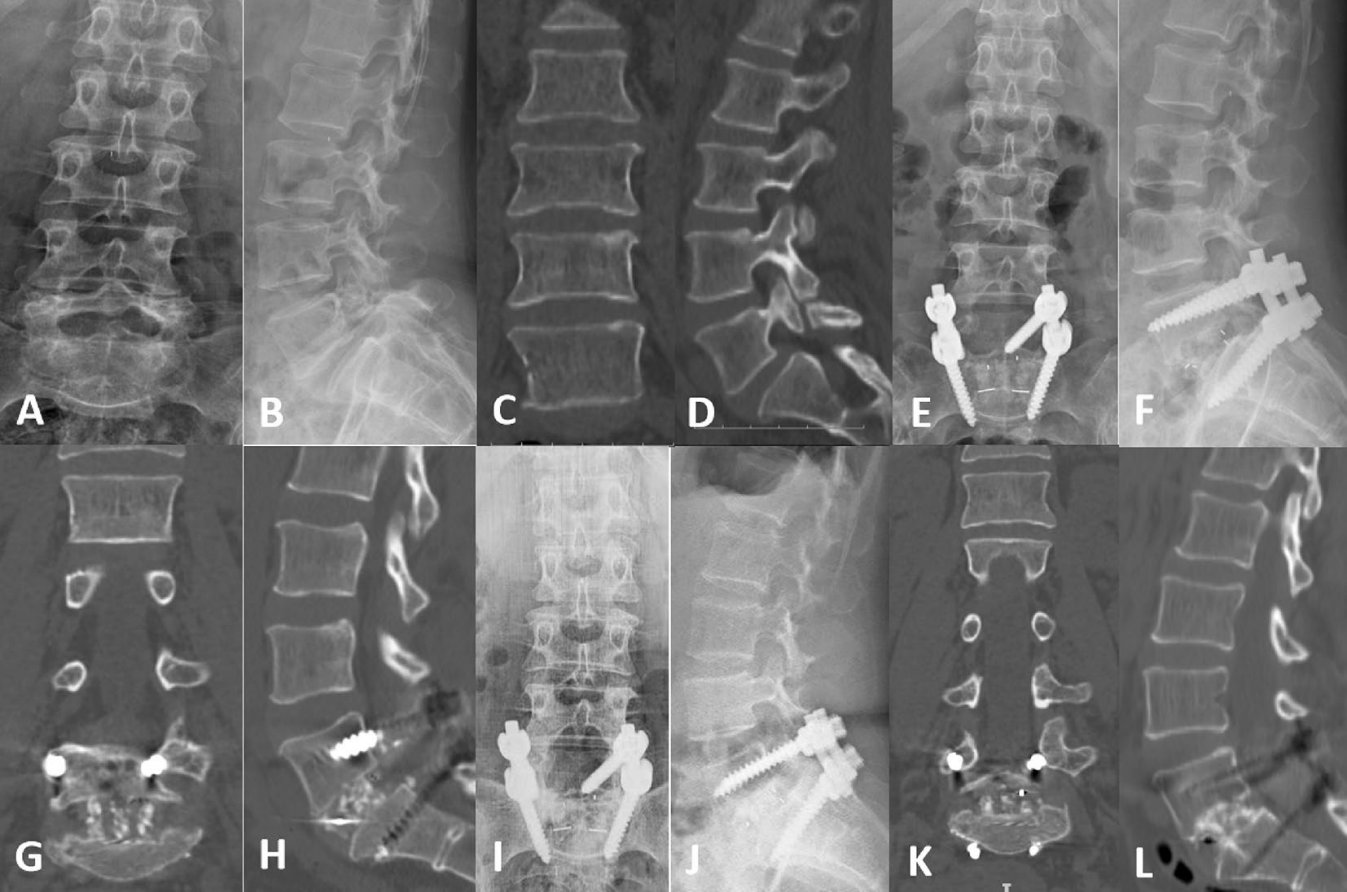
Radiological images of a representative case with CPS. (A–L) A 61-year-old osteoporotic female with isthmic spondylolisthesis in the L5/S1 segments. (A–D) Preoperative X-rays and CT showed forward slip of the L5 vertebra and L5 spondylolysis. (E–H) Postoperative X-rays and CT showed that the internal fixation position was good and that the L5 vertebra was well reset. (I–L) Postoperative X-ray and CT at 24 months after fusion surgery showed that the internal fixation device did not loosen or break, the vertebra did not show slippage, and the intervertebral fusion was good.
